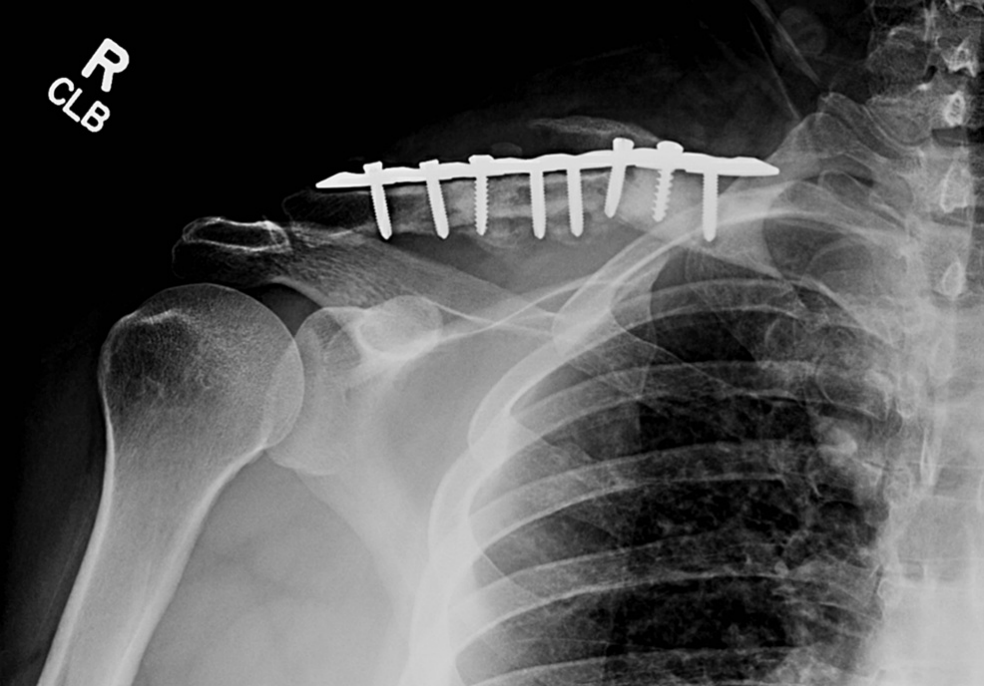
AP radiograph of the right clavicle demonstrating atrophic nonunion, bony erosions, and hardware failure.
